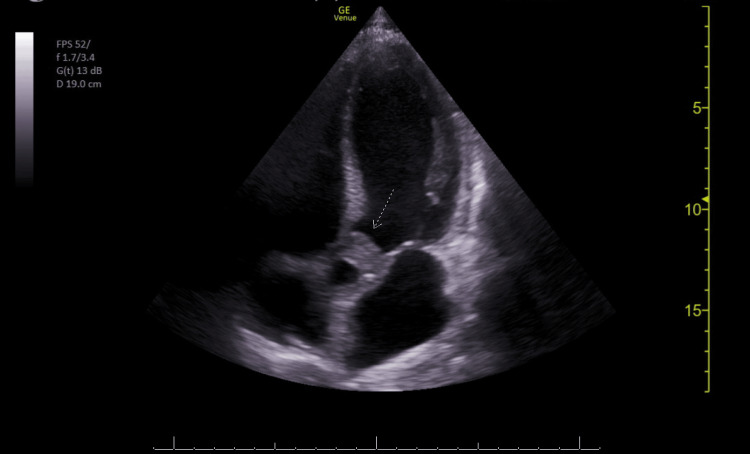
Point-of-care echocardiogram with thickened aortic valve
